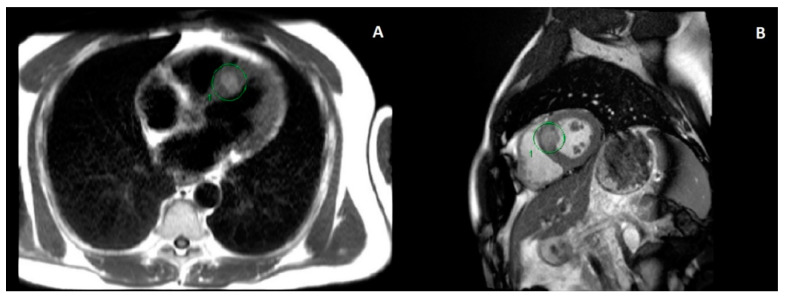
Magnetic resonance imaging (MRI) of the thorax and upper abdomen using gadolinium contrast and cardiac gating. Images from left to right show axial (A) and sagittal (B) views of the metastatic myocardial septum lesion (circled) prior to any treatment.
